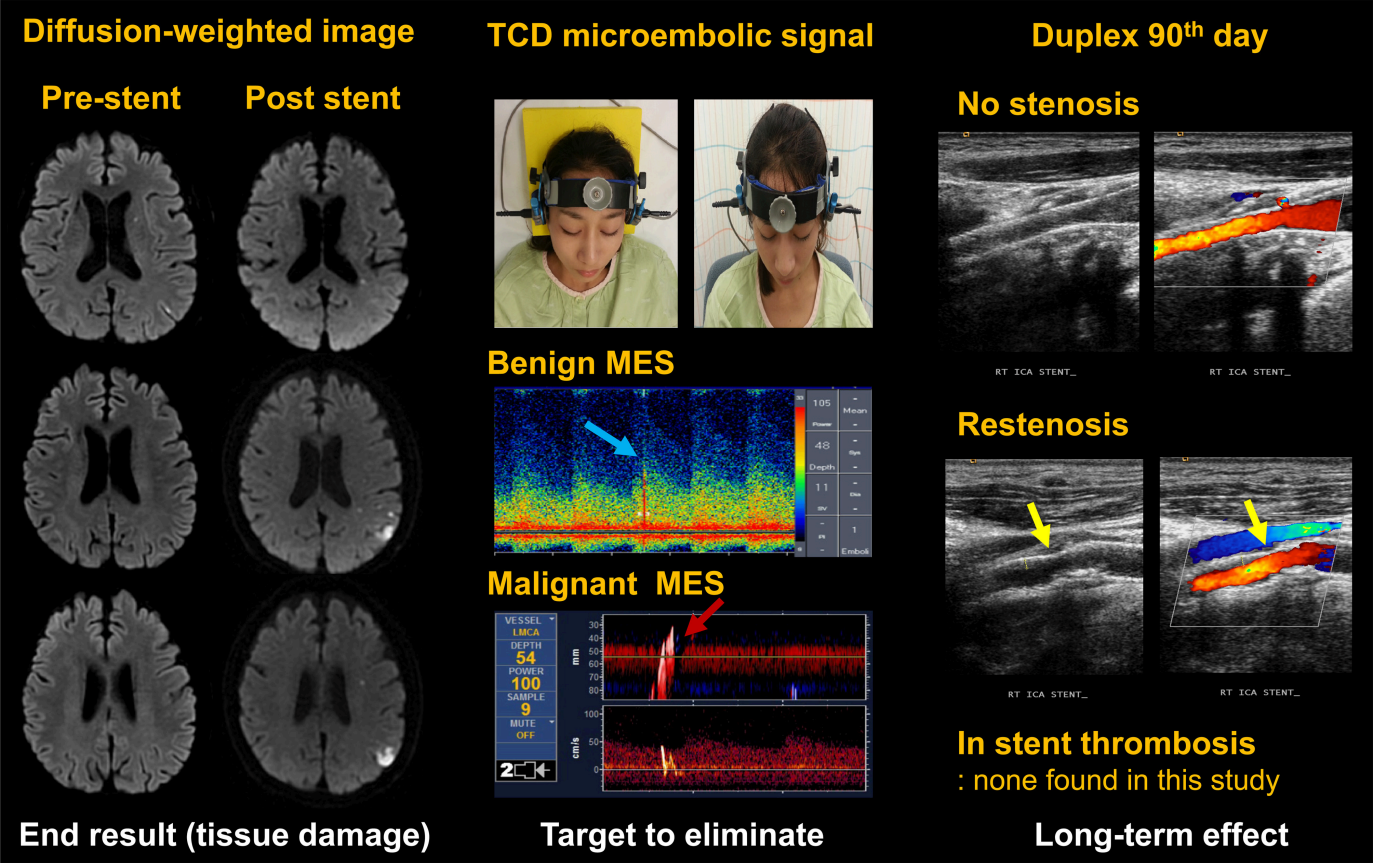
Measurement of laboratory outcomes. TCD, transcranial doppler; MES, microembolic signal. Written informed consent was obtained from the individual appearing in this figure (top, middle).
